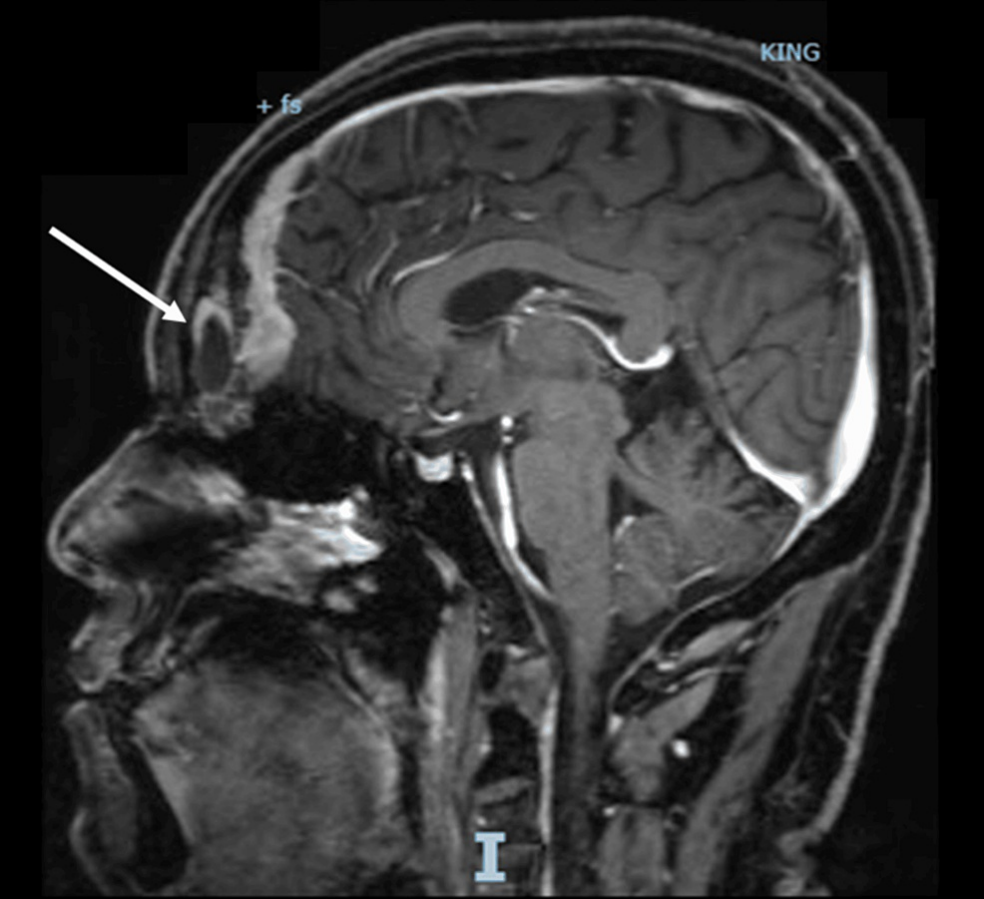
A brain MRI (T2-weighted; without contrast; axial plane) shows a soft tissue lesion located in the midline olfactory groove area. MRI: Magnetic resonance imaging
